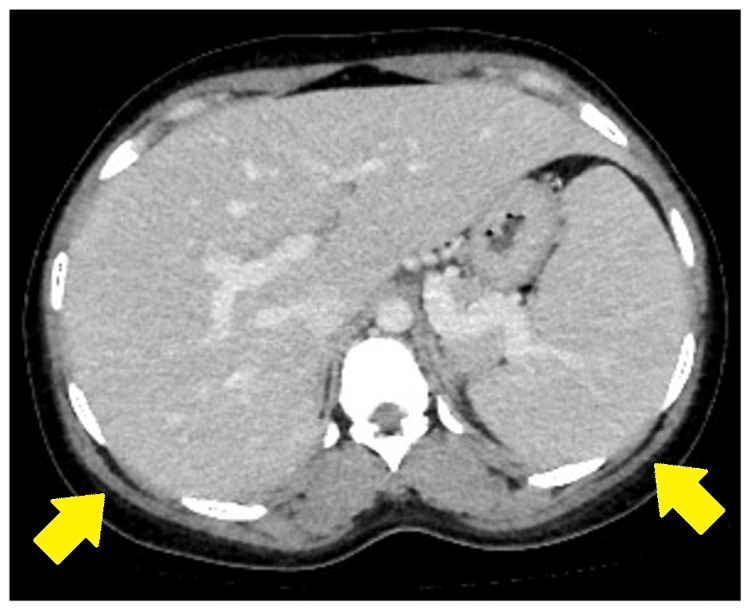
Hepatic enlargement and splenomegaly on contrast-enhanced computed tomography scan.
